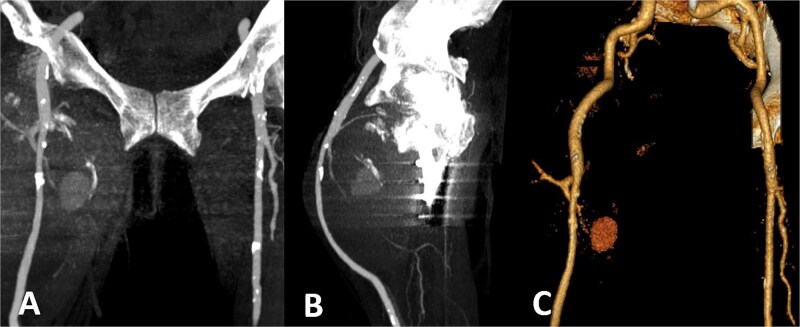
CT angiography. Large pseudoaneurysm (~170 × 107 mm) arising from the profunda femoris artery with partial thrombosis. The mass displaces the superficial femoral artery anteriorly. (A) Coronal CT image; (B) sagittal CT image; (C) three-dimensional reconstruction.
